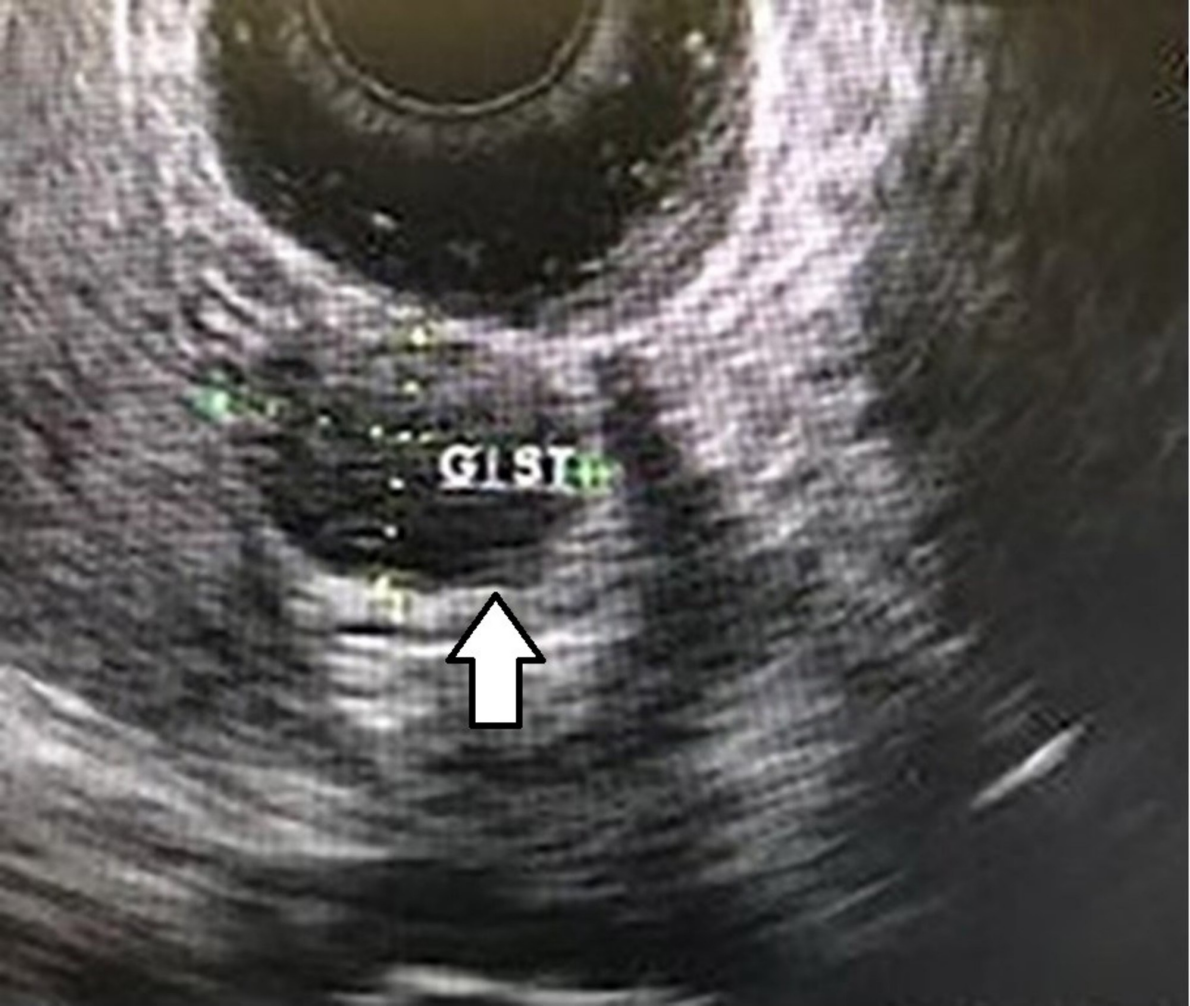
The same 2.4 cm gastric GIST seen on endoscopic ultrasound, shown with arrow. GIST - Gastrointestinal stromal tumor
